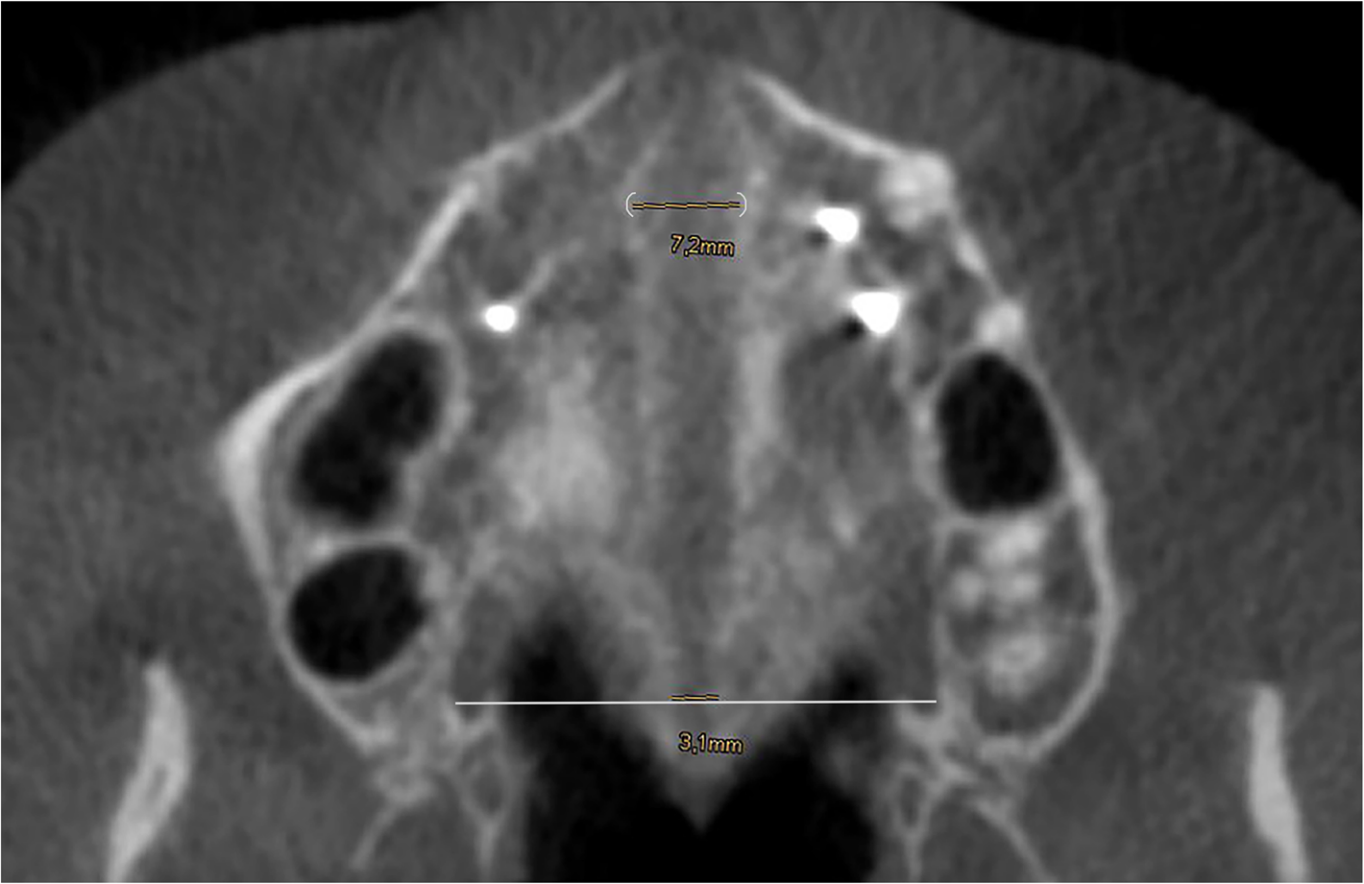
Measurements on CBCT after maximum of non-surgical expansion at the anterior and posterior palate. The nasopalatine foramen (white bracket) and the greater palatine foramina on both sides (white line) were references for measurements
